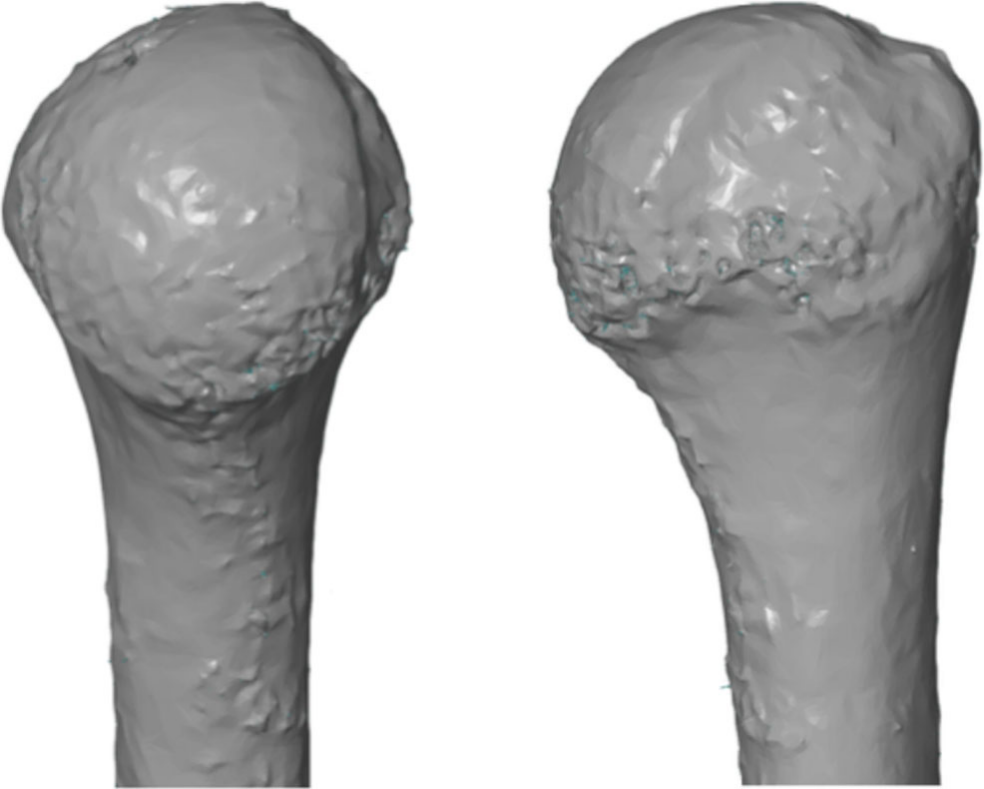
3D-CT reconstruction of humeral head in GOM Inspect (V8) software
